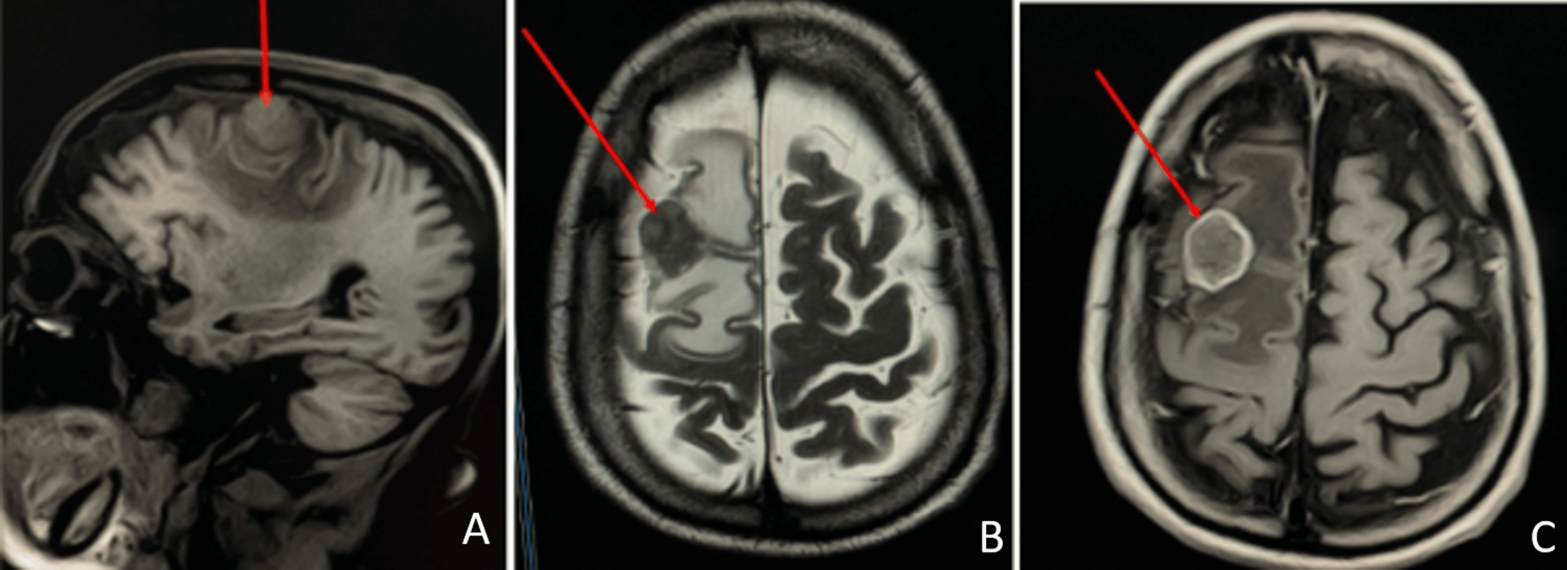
Brain MRI (A) Sagittal view showing a well-defined nodular lesion (red arrow) observed in the precentral gyrus of the right frontal lobe, with signal intensity isointense to the gray matter. (B) Axial T1-weighted image without contrast, confirming the isointense signal of the lesion relative to the cerebral cortex; red arrow shows the lesion. (C) Axial T2-weighted image; the red arrow shows the hypointensity of the lesion, surrounded by a broad area of hyperintensity consistent with vasogenic edema, causing effacement of adjacent sulci and fissures. Postcontrast sequences demonstrate peripheral ring enhancement and restricted diffusion, suggestive of residual tumor. The lesion measures approximately 19 × 16 mm in the axial plane MRI: magnetic resonance imaging
